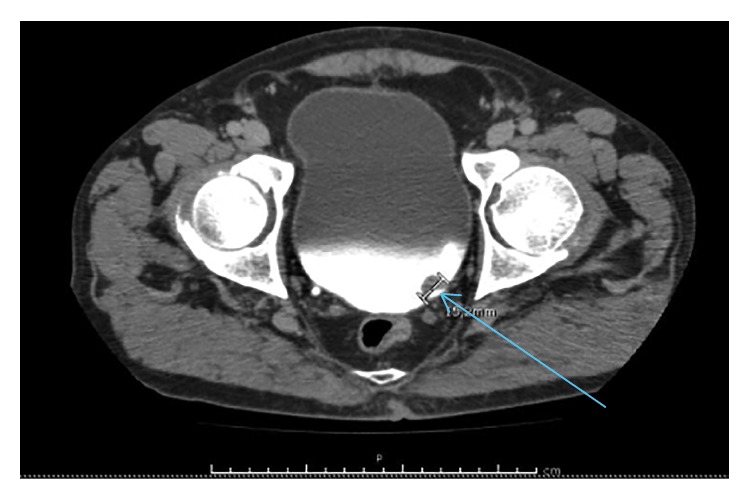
Sagittal section Uro-CT scan showing proliferative lesion left ureteral meatus (arrow).
